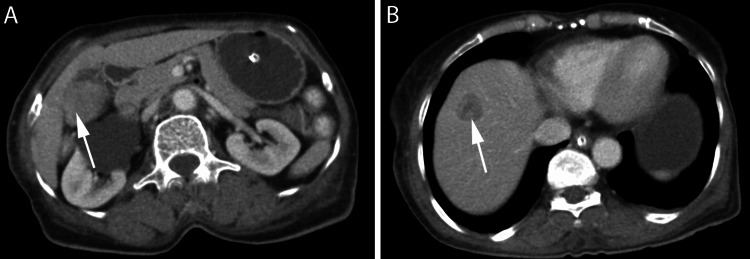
Contrast-enhanced CT revealing enhancing polypoidal soft tissue density lesion involving the gallbladder (A) with solitary liver metastasis (B).
